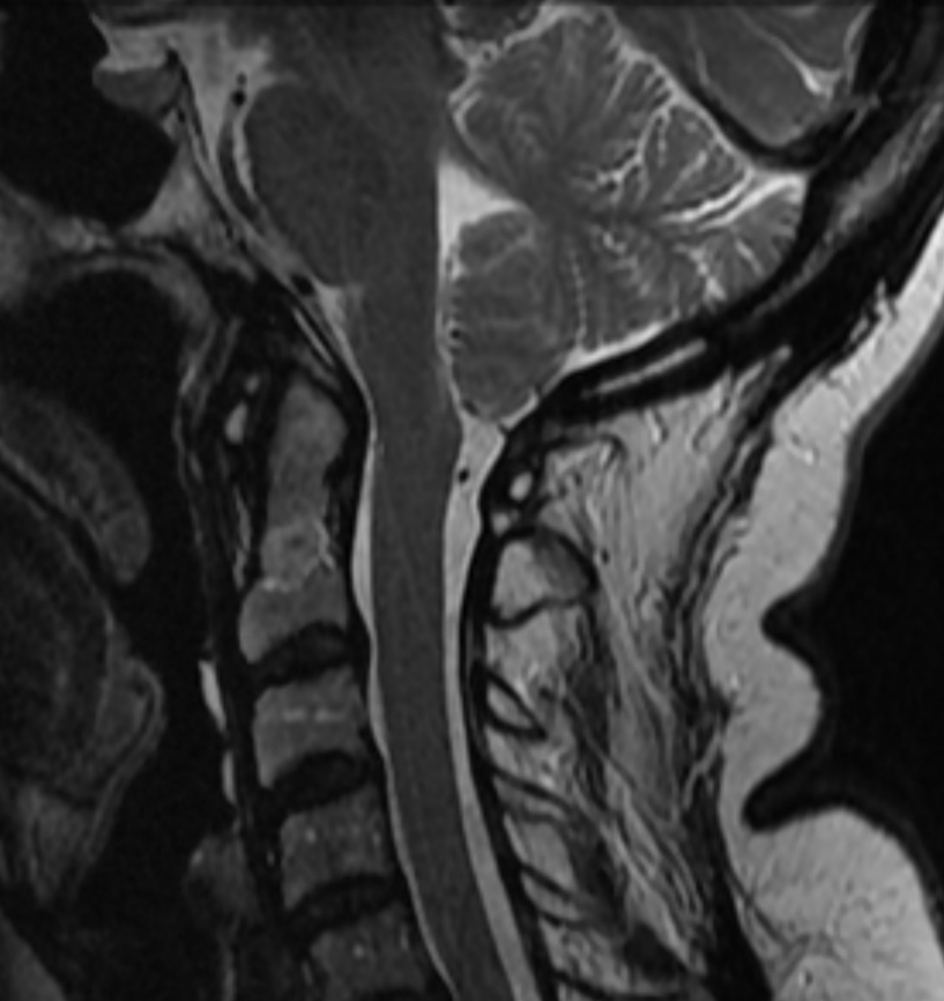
Sagittal section *T*_2_ weighted MR image of the upper cervical spine and posterior cranial fossa demonstrating normal tonsillar position and morphology.
